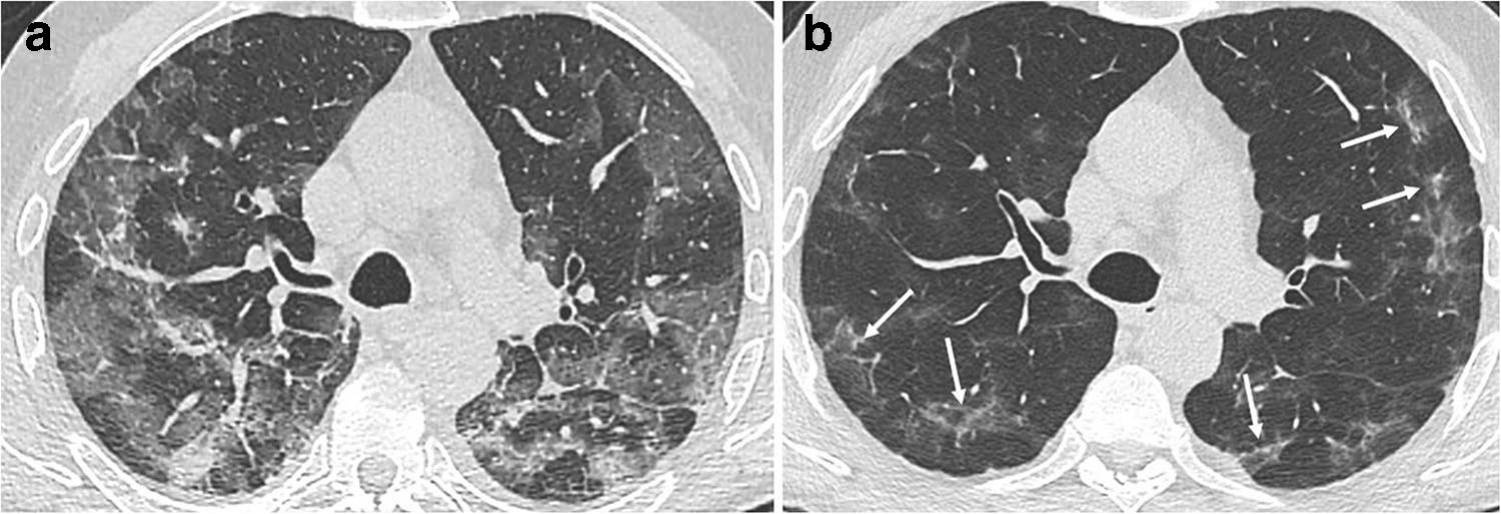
CT features at baseline and 3-month follow-up, in a 60-year-old man with initial medium severity (10-day oxygen supplementation). a Baseline CT showing bilateral peripheral ground glass opacities (GGOs). b 3-month CT follow-up. GGOs significantly improved. Some areas of linear consolidation parallel to the pleura are demonstrated (arrows), consistent with late phase organizing pneumonia. Mild deformation of the left major fissure is noted, but there are no formal signs of fibrosis such as traction bronchiectasis or honeycombing
